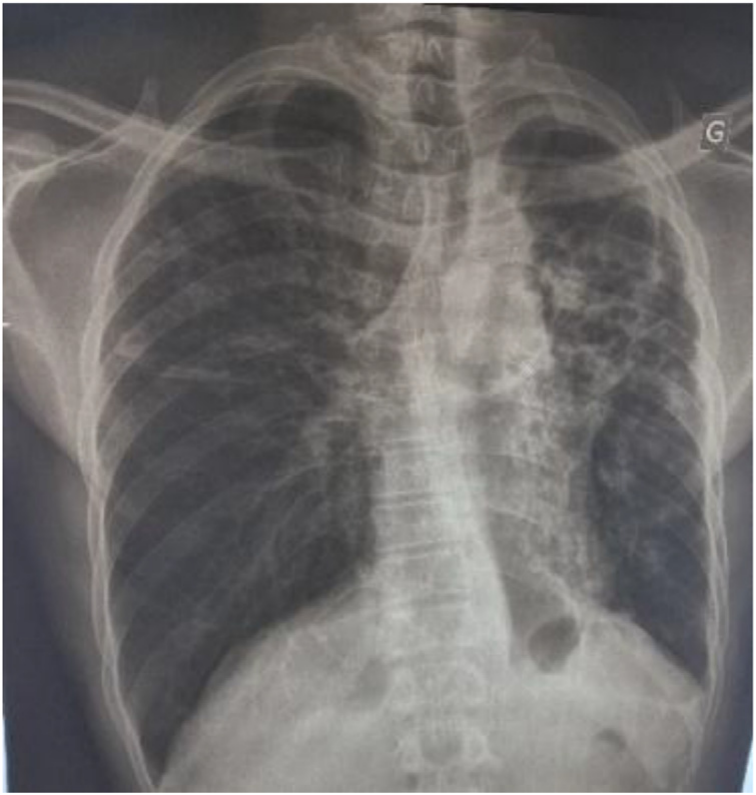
Chest X-ray showing left-sided cavitary and alveolar lesions at the time of diagnosis in case 2.
